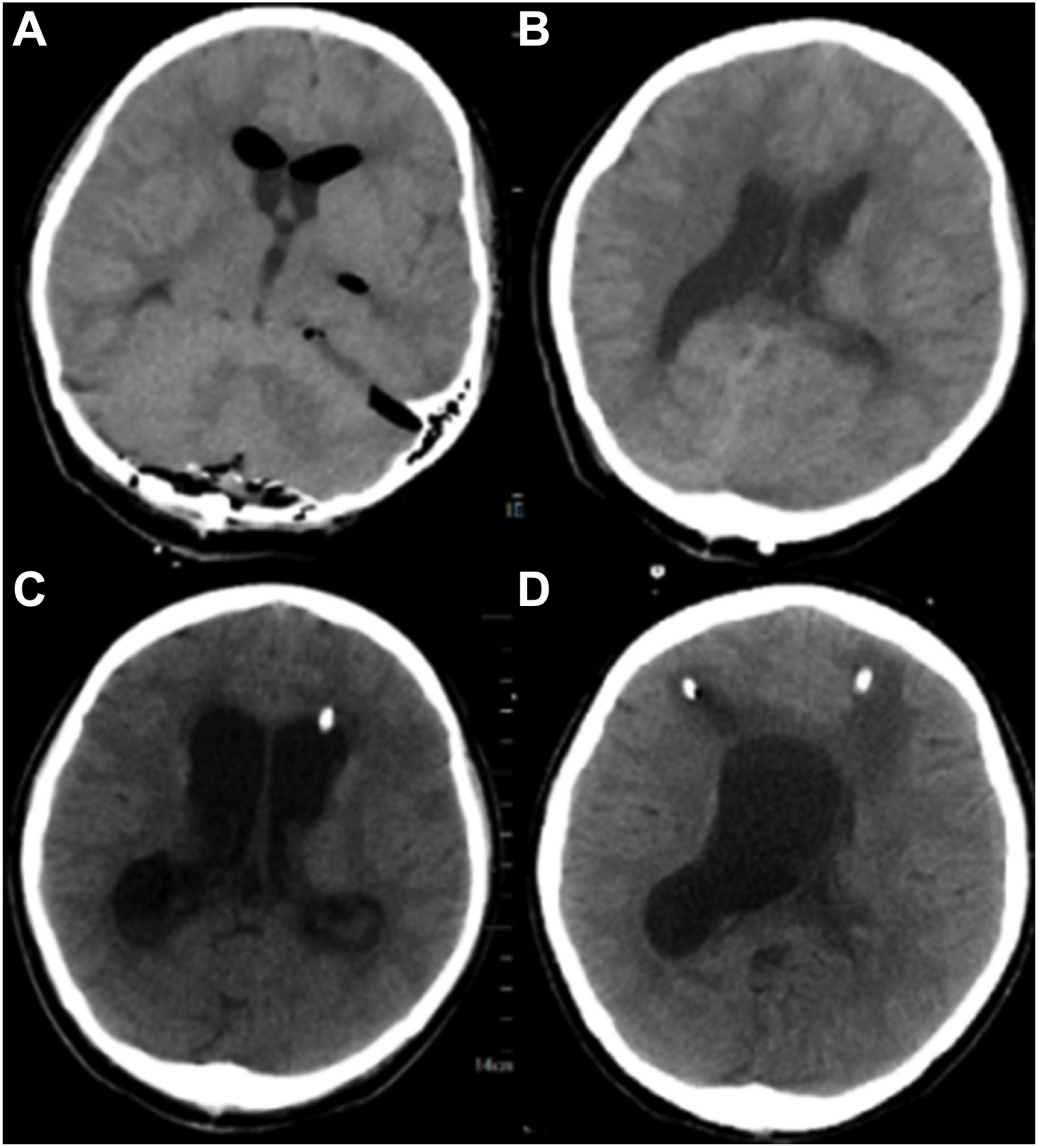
Brain CT of the patient in different stages of the disease. (A, B): Postoperative CT of the patient. (C) On the 17th postoperative day, the patient's head CT after left ventriculocentesis. (D) On the 27th postoperative day, the patient's head CT after left ventriculocentesis.
